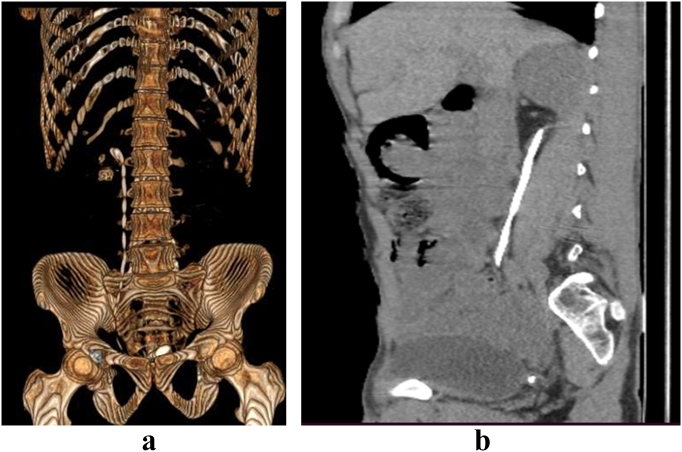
A: CT scan 3D image of Stent retained for 15 years shows significant encrustation, right nephrolithiasis and associated bladder calculus. B: CT scan sagittal view.
